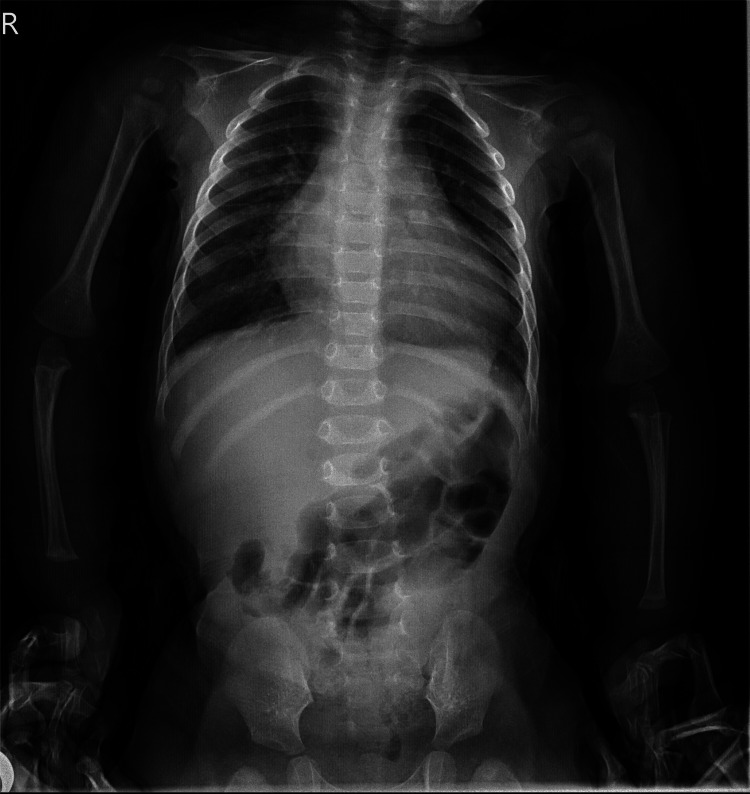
Anteroposterior chest radiograph showing marked cardiomegaly with an increased cardiothoracic ratio, reflecting significant cardiac enlargement.
